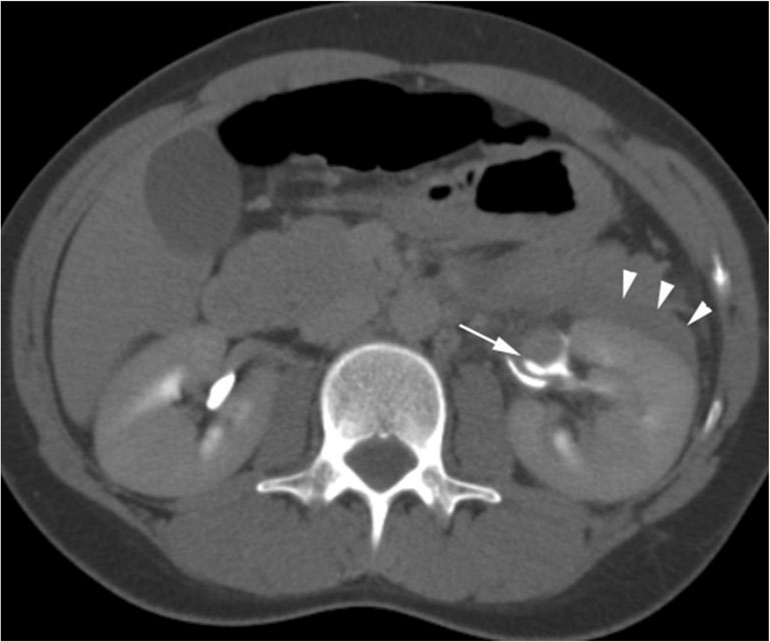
A delayed-phase CT shows a left renal pelvis with extravasation of urine anterior to it (*arrow*). Note the unopacified urine in the perinephric space (*arrowheads*)
